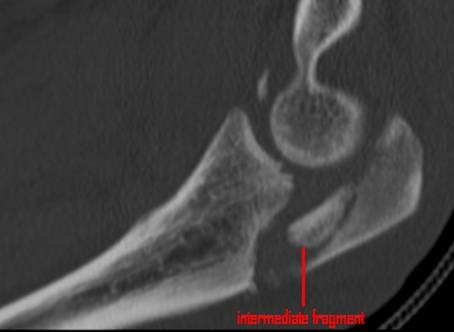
Post-traumatic CT scan shows closed olecranon fracture classified as Mayo type IIIb fracture.
